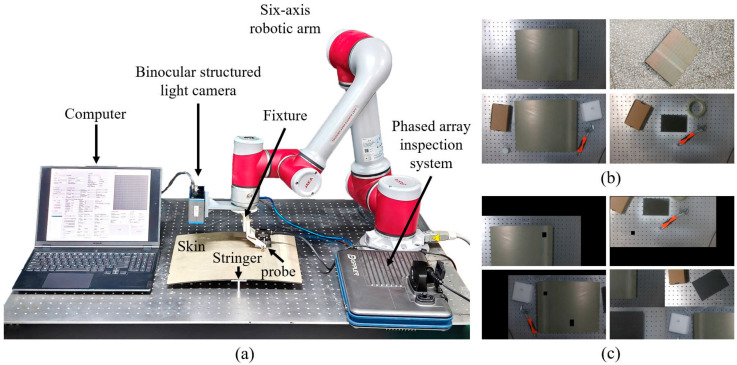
Experimental platform and dataset for robotic arm vision-guided ultrasonic automated inspection: (a) Experimental platform, (b) visible light dataset, (c) data augmentation.
